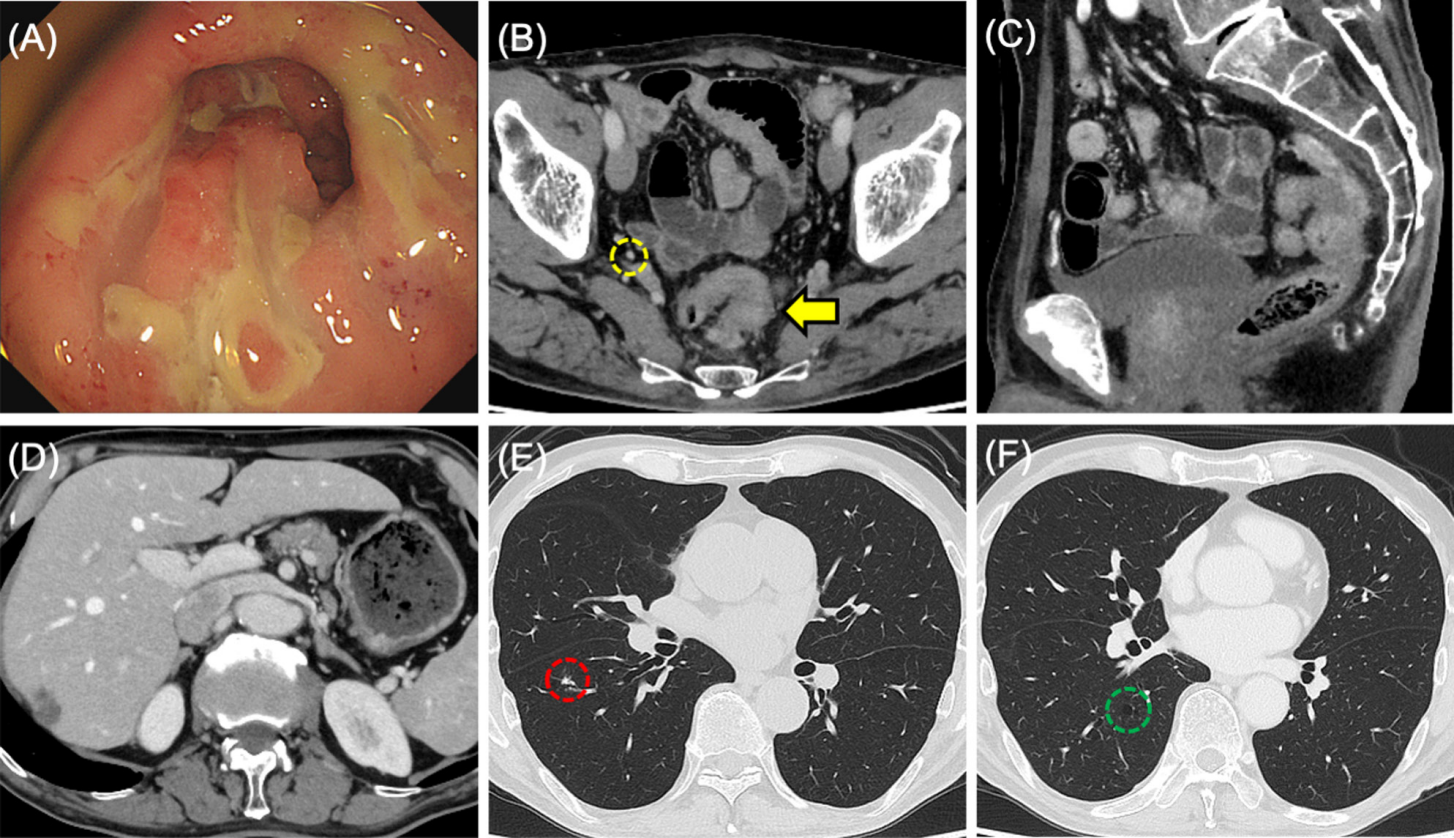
Preoperative diagnostic images after chemotherapy. (A) Colonoscopy showing findings that suggest tumor shrinkage with narrow patency of the rectal lumen. (B–F) Computed tomography showing a shrunk lateral lymph node in the right internal iliac region (yellow dotted circle) with a reduction of the primary rectal tumor (yellow arrow) (B, C). Additionally, the size of the metastatic liver tumor decreases to 1.7 × 1.2 cm (D), and the metastatic lung tumor becomes a radial scar (red dotted circle) and vacuolization (green dotted circle) (E and F, respectively).
